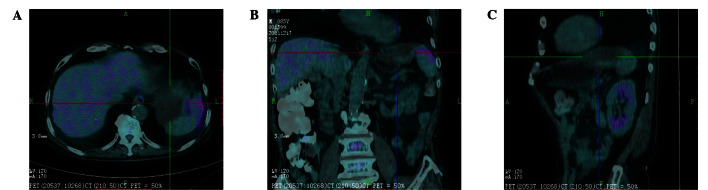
(A) Transaxial, (B) coronal and (C) sagittal PET/CT images showing an uneven, rim-shaped FDG-uptake pattern, rather than a global pattern at the tumor periphery. In the false-colour images, gray indicates no FDG-uptake, blue indicates weak FDG-uptake, purple indicates moderate FDG-uptake and red indicates marked FDG-uptake. PET/CT, positron emission tomography-computed tomography; FDG, fludeoxyglucose (^18^F).
